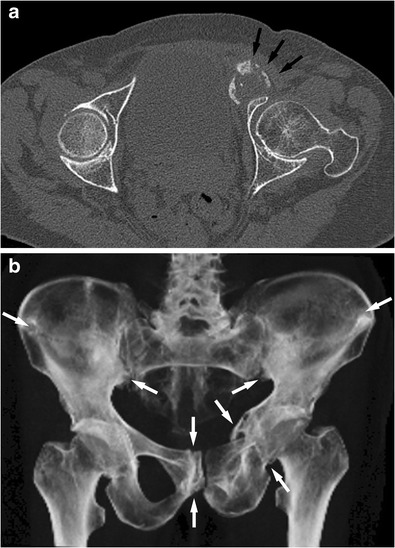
CT of the pelvis of an elderly female with multiple stress fractures due to osteoporosis. The axial images of the fractures could be mistaken for an aggressive destructive process (a, arrows) showing an apparent expansile bone lesion anterior to the acetabulum. However a volume-rendered image (b) of the pelvis shows multiple and partly symmetrical fractures typical for severe insufficiency fractures. Fractures of the iliac blades, the right os pubis and left pubic rami can be seen (see arrows). The axial images in isolation may be difficult to interpret; 3D reformats and volume rendering help in image interpretation
